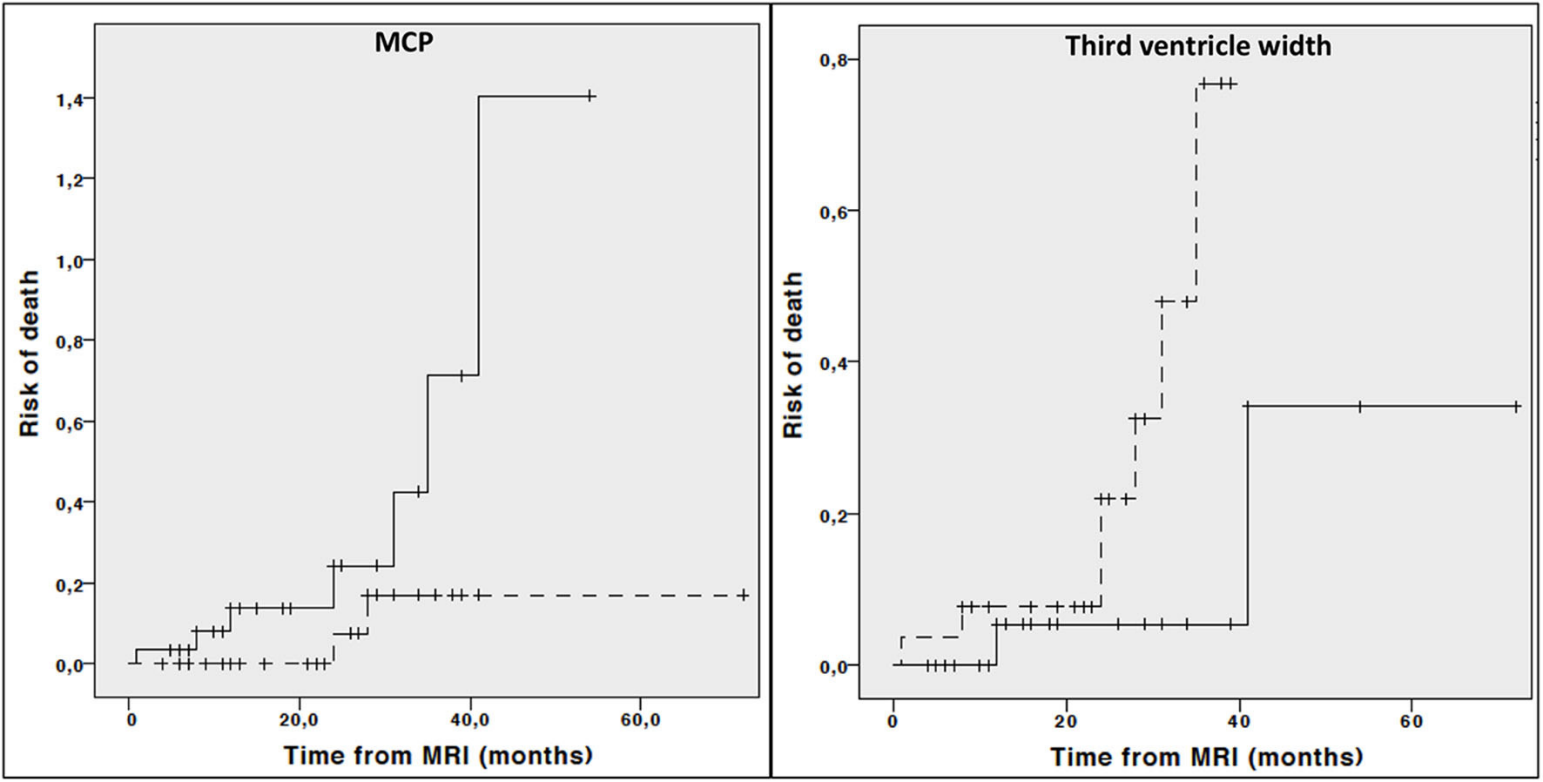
Cumulative risk of death by each MRI parameter (less atrophy vs. more atrophy). Only curves with significant log-rank test at 0.05 level are reported. Dotted line: values above median; Continous line: values below median.
